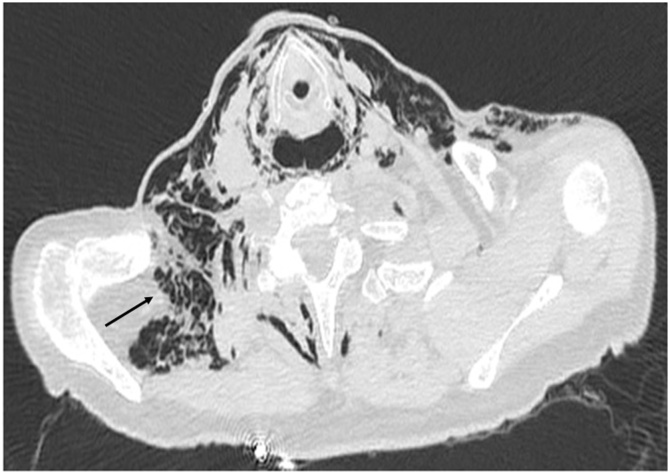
Chest CT shows subcutaneous emphysema.
